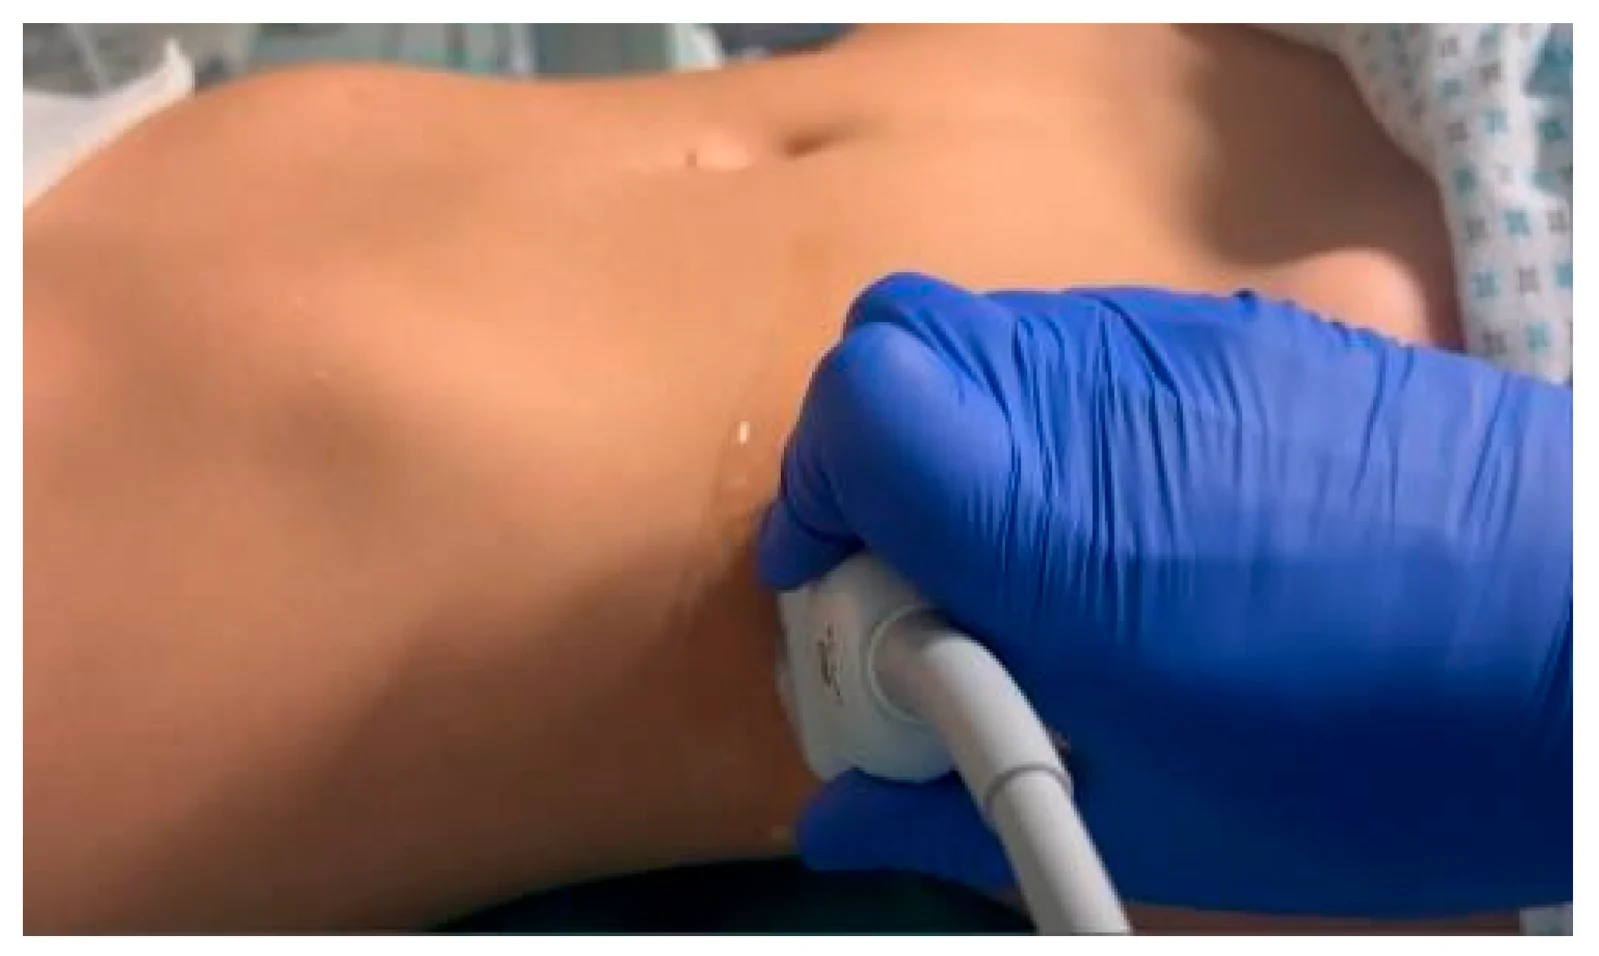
Application of the ultrasound probe.
